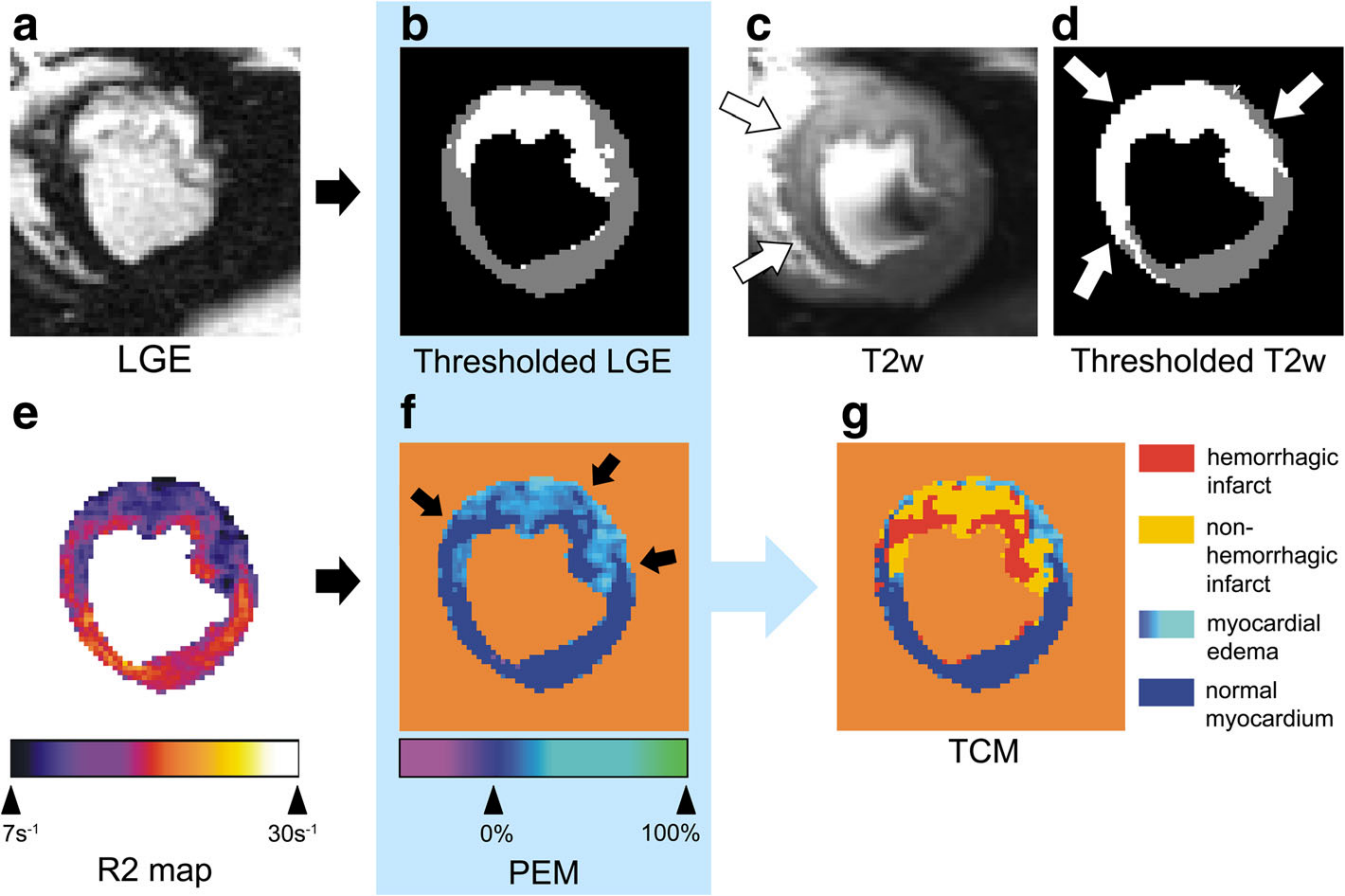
Corresponding short-axis MR images and post-processed parametric maps are shown. The MI region appears enhanced in the LGE image (a). Thresholded LGE image shows the irreversibly injured region as a *white area* (b). T2-weighted image (c) and corresponding thresholded T2-weighted image (d) highlight the increased signal in the MI region and septum (*white arrows*). Note that the crude method of thresholding T2-weighted images overestimates ME and is unable to differentiate regions with a varying extent of ME. Therefore, T2-weighted imaging cannot differentiate hemorrhagic from non-hemorrhagic MIs. PEM (f) calculated from the R2 map (e) shows the AAR (*black arrows*). There is an apparent lack of ME in the center of the MI due to cancelling effect by acute hemorrhage. TCM (g) generated from b and f defines the ME region surrounding the non-hemorrhagic part of the necrotic tissue, and the hemorrhagic region in the center of the MI. Corresponding TTC pathology is shown in Fig. 3
